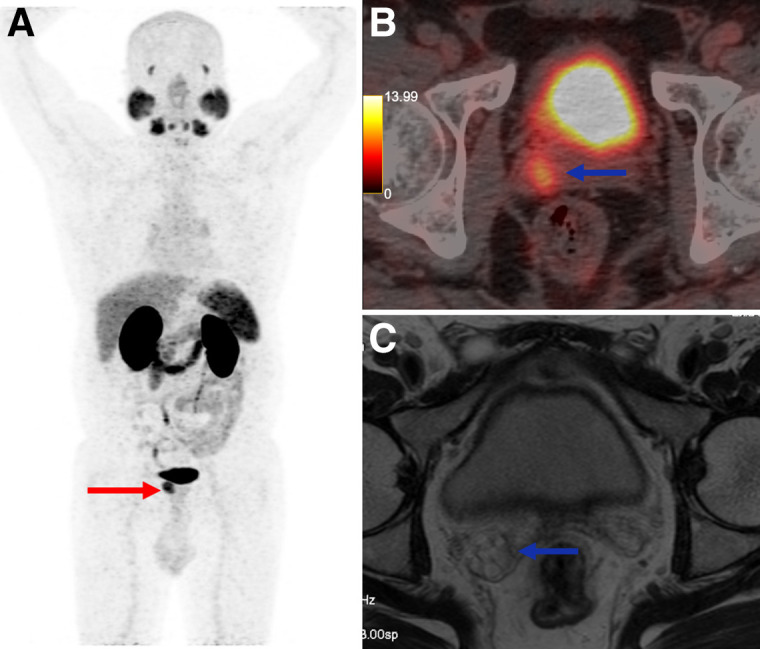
A 55-y-old man presenting for initial staging of PCa. (A) ^68^Ga-PSMA-11 PET/CT maximum-intensity projection shows PSMA-expressing lesion involving prostate (red arrow), with PSMA avidity in right seminal vesicle invasion (blue arrow). (B) PET/CT axial image shows right seminal vesicle invasion (blue arrow). (C) However, MRI at same topography shows no seminal vesicle invasion. Pathology analysis confirmed ^68^Ga-PSMA-11 PET/CT–positive results.
